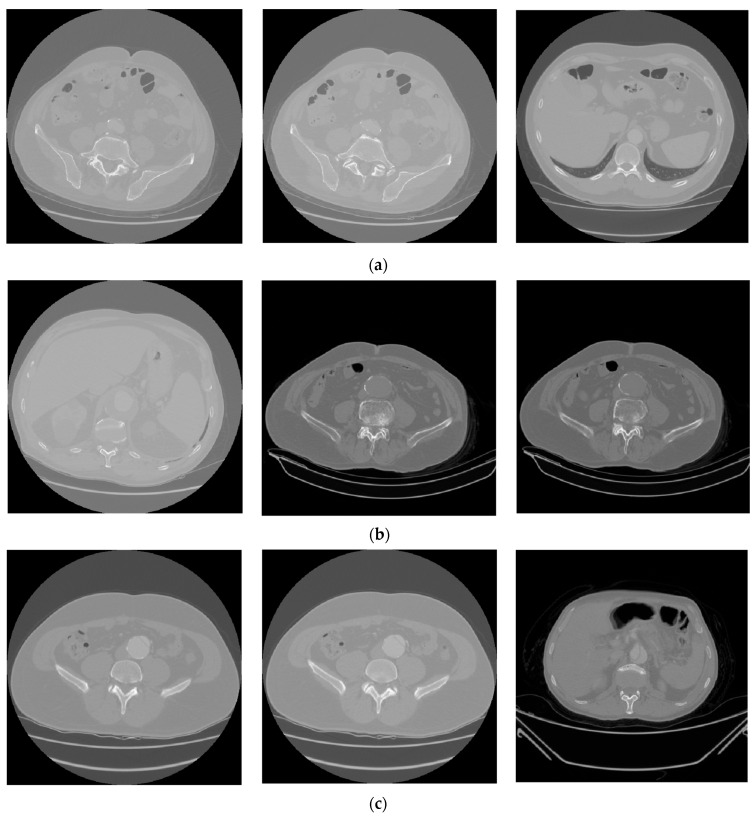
Some abdominal CT images of (a) no disease, (b) AAA, and (c) AAD in the dataset.
